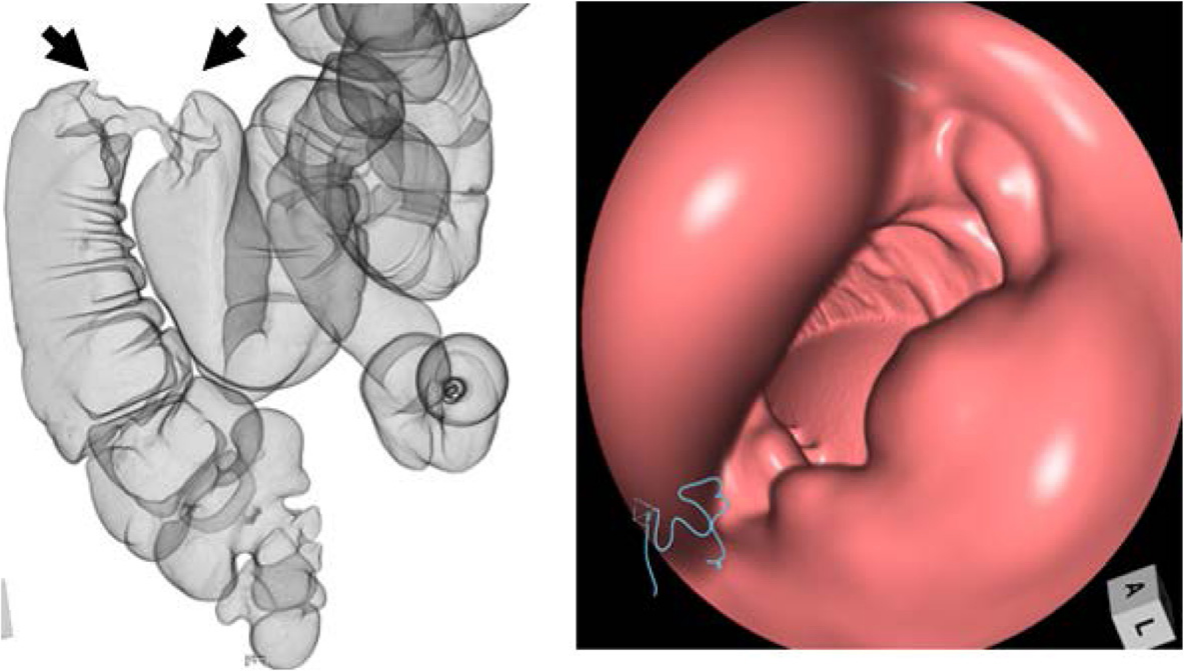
Computed tomographic colonography revealed the apple core sign in the hepatic flexure (*black arrows* show), without any evident tumor in the proximal colon
